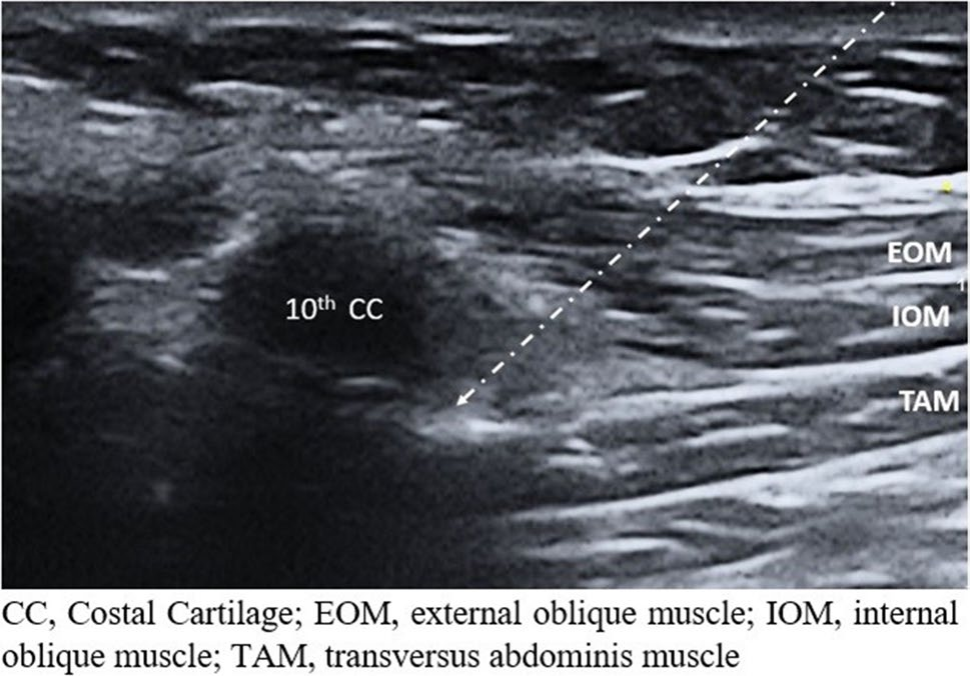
Ultrasound image of M-TAPA block application
